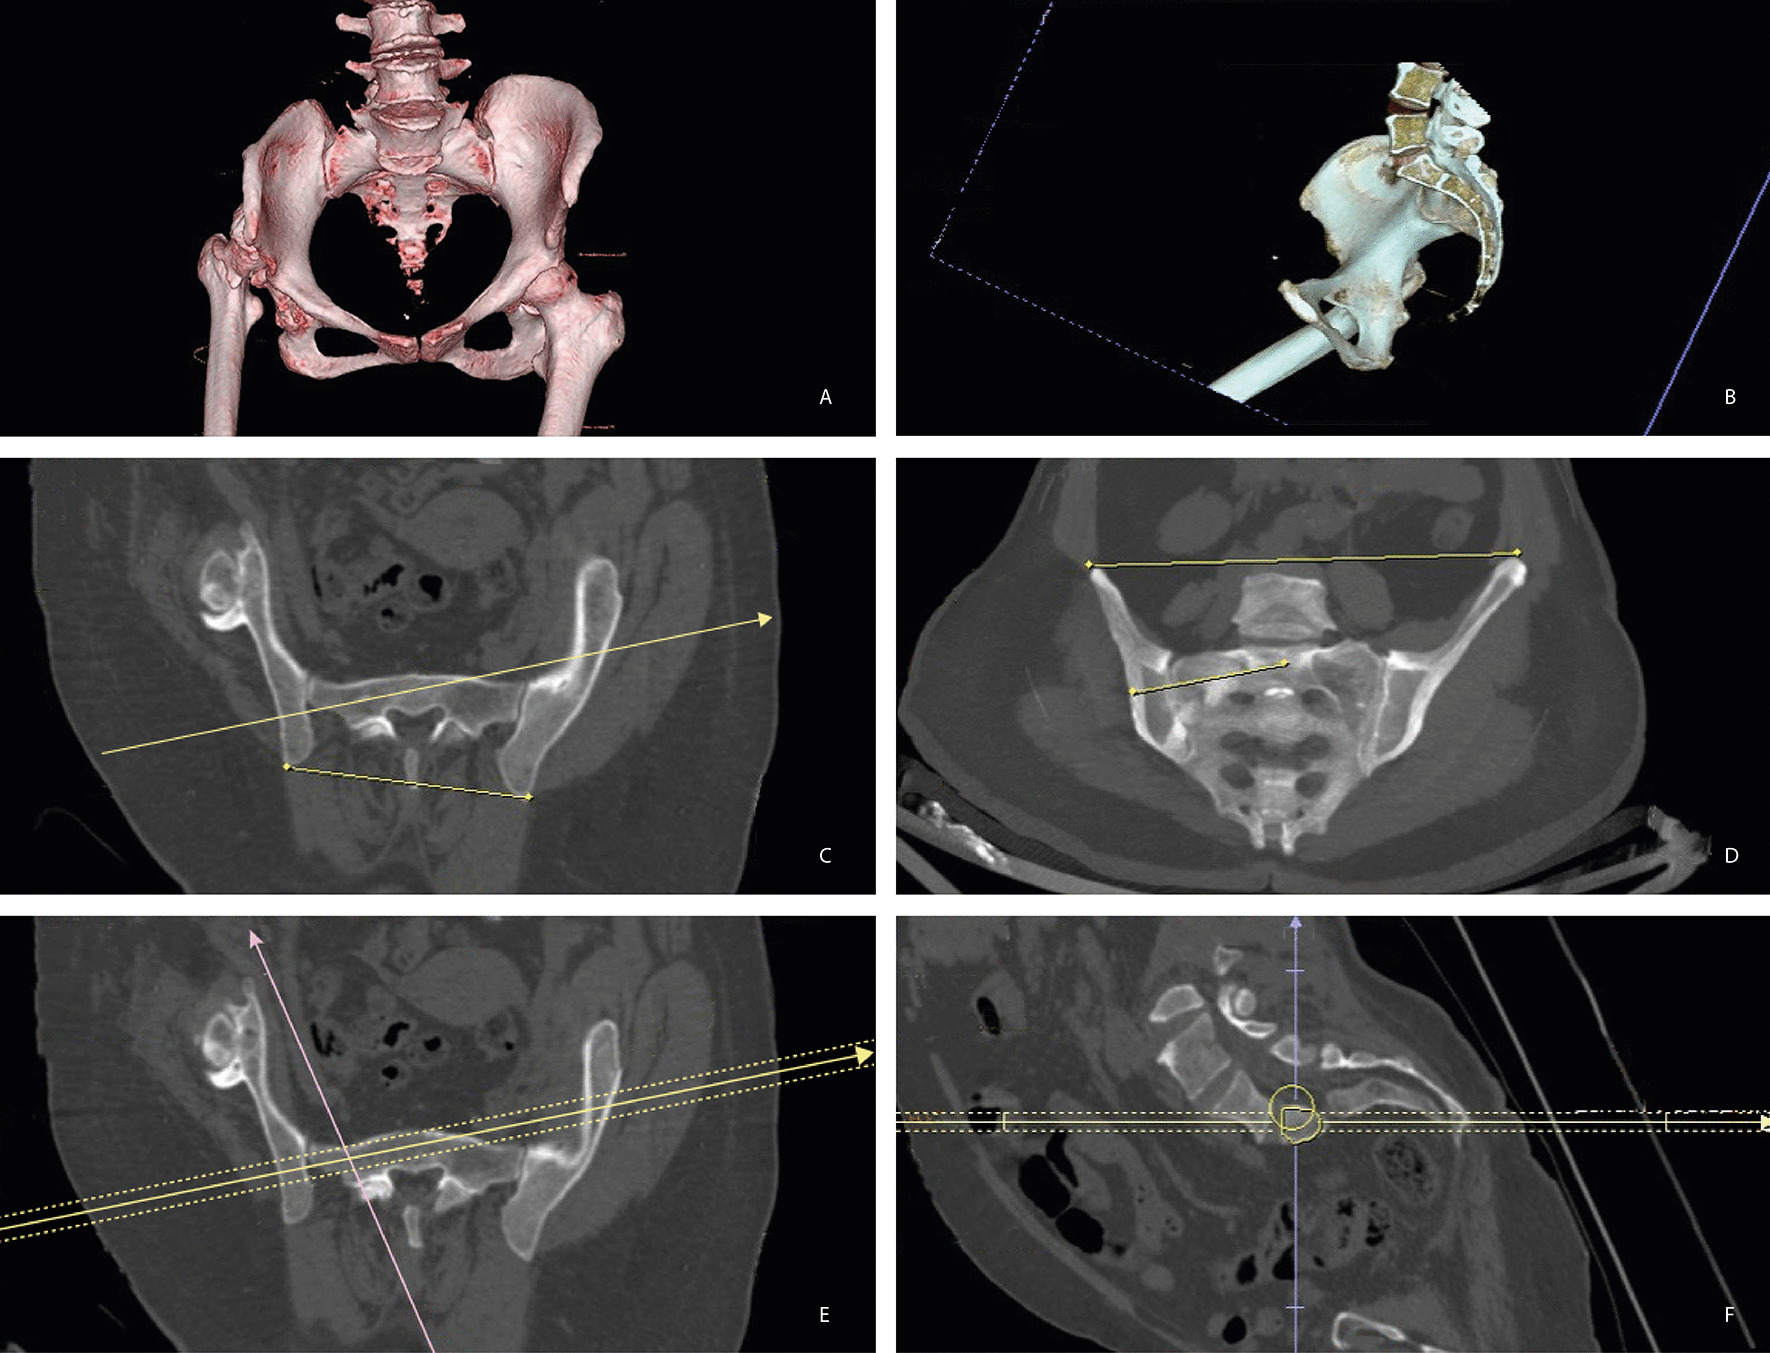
3D pelvic CT reconstruction images were analyzed in a 55-year-old patient with right unilateral DDH. A Bilateral sacral dysmorphism, DDHon the right side are seen. B More than half of the S1 body located above the iliac cortical density on the right. C ve D axial and coronal reformats made perpendicular to the S1 and S2 sacral osseous corridors. Axial and coronal angulations of the first and second sacral segments were measured. E The maximum length of a 10 mm diameter osseous corridor measured. F Sagittal computed tomography image showing the cross-sectional area of the safe zone
